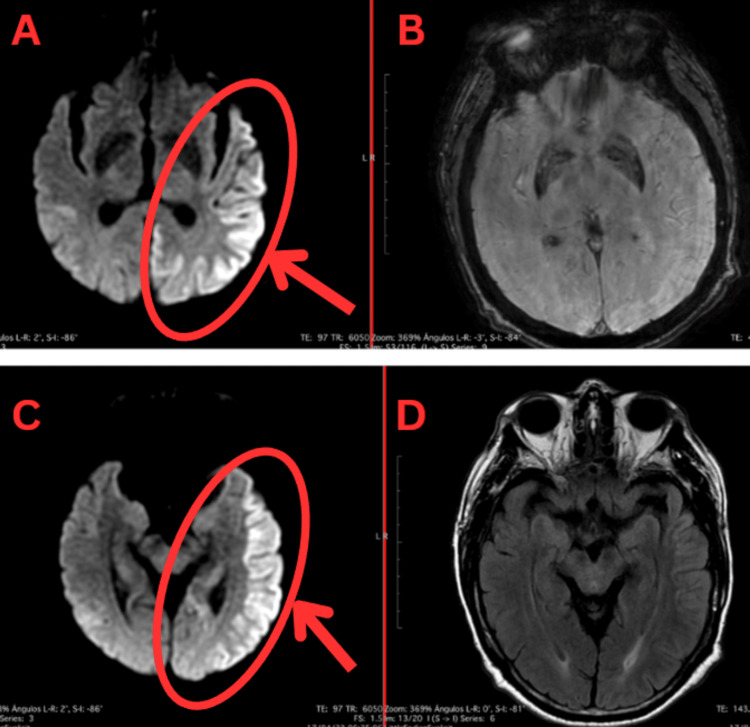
Third axial brain MRI: diffusion-weighted imaging (DWI), SWI, and fluid-attenuated inversion recovery (FLAIR) sequences Panels A and C (DWI sequences): Inflammatory changes in the left temporo-parietooccipital cortex and right parietal and temporal regions, with increased affected areas compared to the previous study. Panels B and D (SWI and FLAIR sequences) do not reveal any associated lesion.
